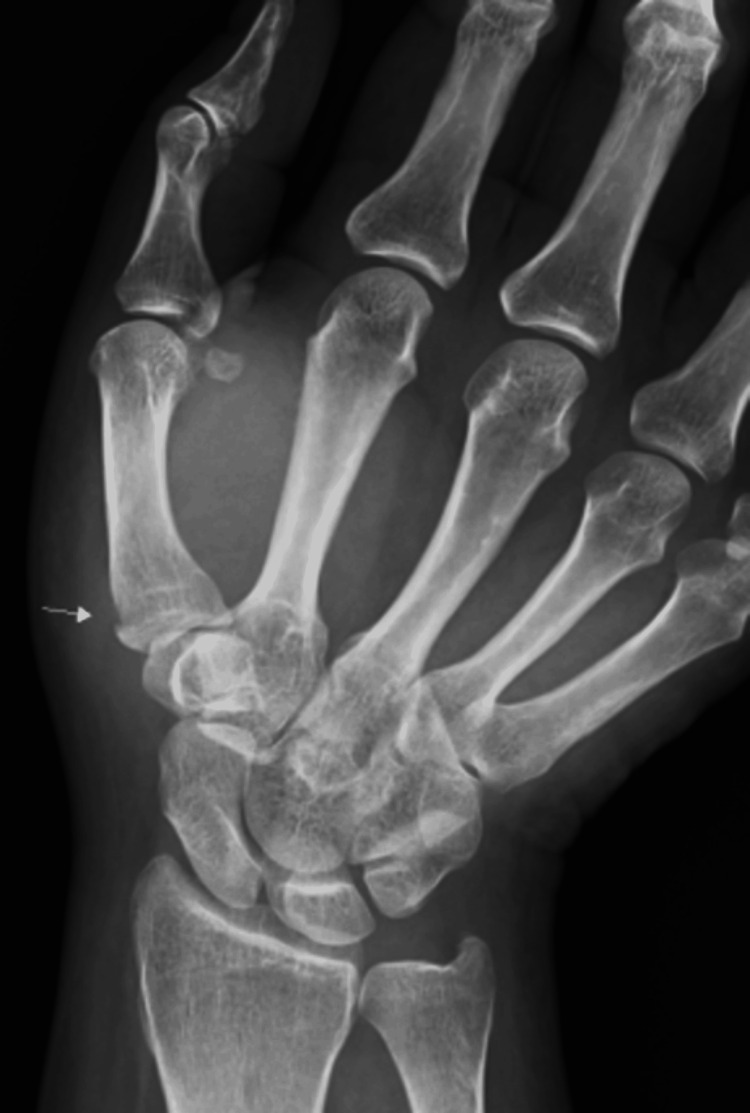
Plain radiograph of the right hand in Case 2 The white arrow indicates osteolytic changes at the base of the first metacarpal bone
